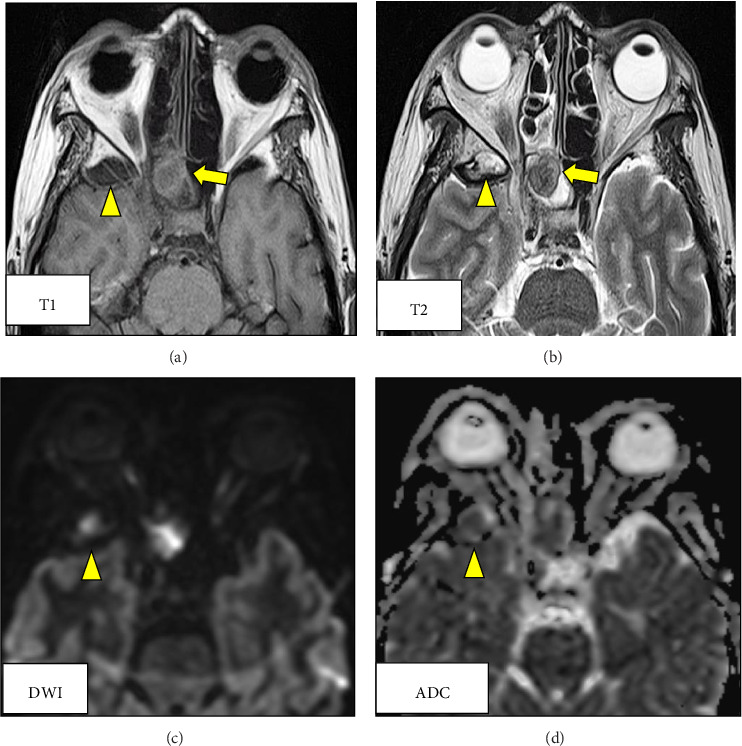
Magnetic resonance imaging scan showing an epidural hematoma in the right middle cranial fossa (yellow arrowhead) and findings indicative of mycosis fungoides the right sphenoid sinus (yellow arrow) (a, b). T1-weighted imaging showing high- and low-intensity areas in the peripheral and internal regions of the right sphenoid sinus, respectively (a). T2-weighted imaging showing low-intensity areas of the right sphenoid sinus on (b) (yellow arrow). T2-weighted imaging (b) and diffusion-weighted imaging (c) showing high intensity, and the apparent diffusion coefficient (ADC) (d) shows low intensity of center (yellow arrowhead).
